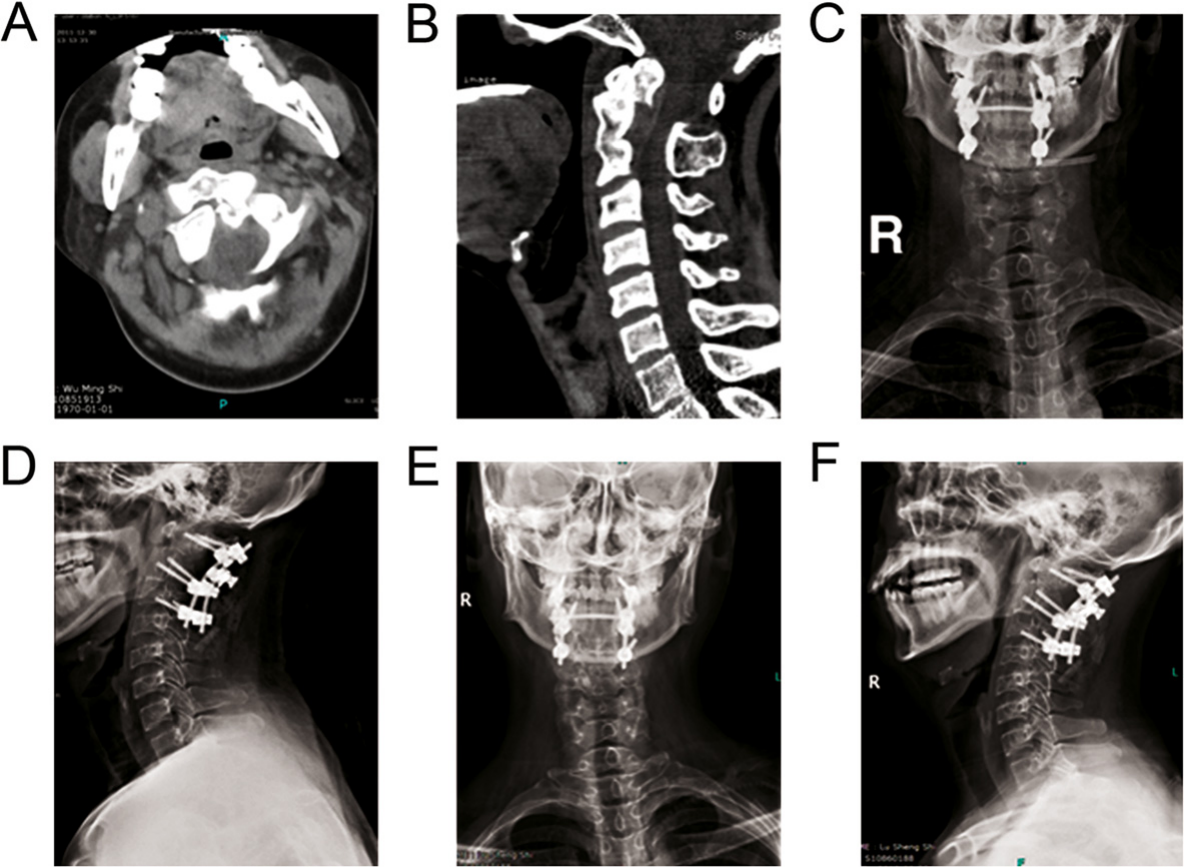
A 43-year-old male patient developed pain symptoms and paralysis of two lower extremities by traffic accidents. CT scanning indicated fractures of the odontoid process of the axis (A), combined with C1–2 and C2–3 dislocation (B). Anteroposterior (C) and lateral (D) cervical spine X-ray scanning showed excellent reduction after posterior C1–2 cervical pedicle screw and C3 lateral mass screws fixation. At 1 year after operation, the anteroposterior (E) and lateral (F) cervical spine X-ray scanning suggested bone fusion and no displacement of the implant.
